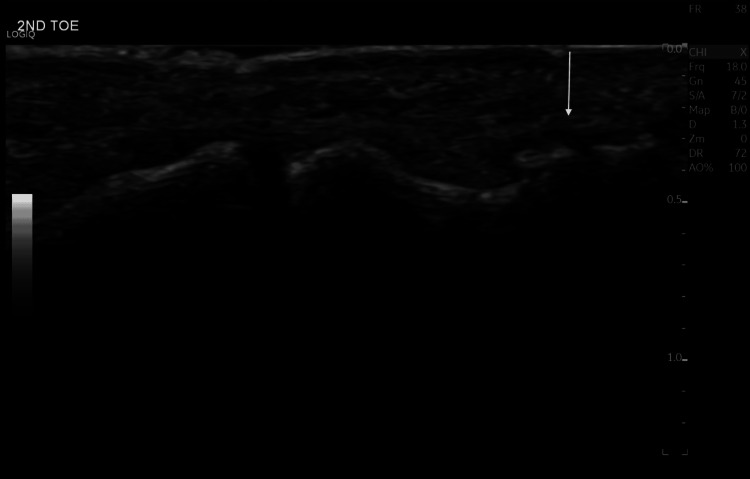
Ultrasound of the second toe of the right foot (dorsal aspect) in 2024. There are no obvious detectable masses, fluid collections, or abnormal flow signals seen in this ultrasound. The interphalangeal joint appears normal and is well-defined, with smooth cortical margins as labeled by the arrow.
